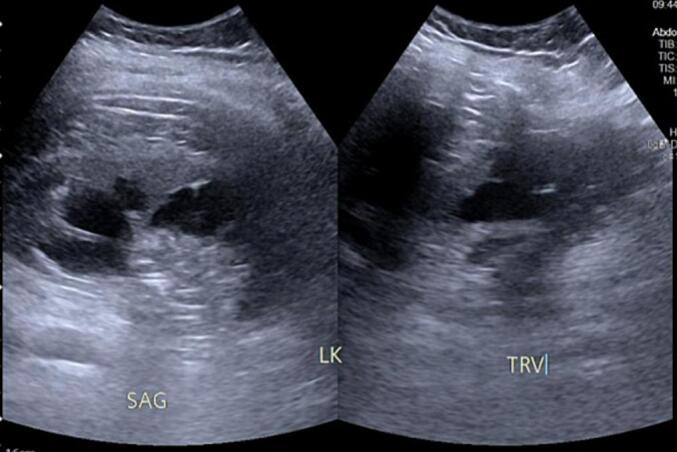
Ultrasound of the patient after 2 months.
